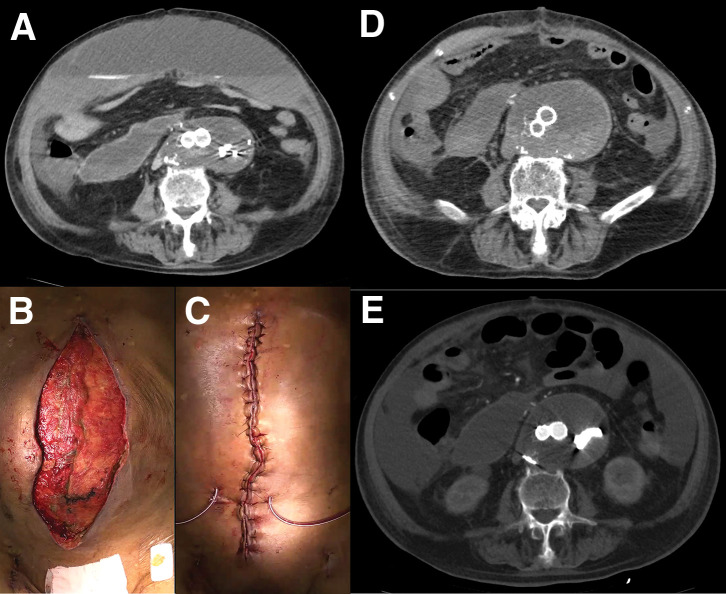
Wound closure following fascial release. Plain CT scan showing fluid accumulation between the rectus sheath and abdominal wall (A). Wound appearance after gauze packing, and closing abdomen (B, C). Post-closure CT scans, including a plain scan (D) and contrast-enhanced scan (E), confirmed the absence of bowel necrosis and abdominal wall hernia.
